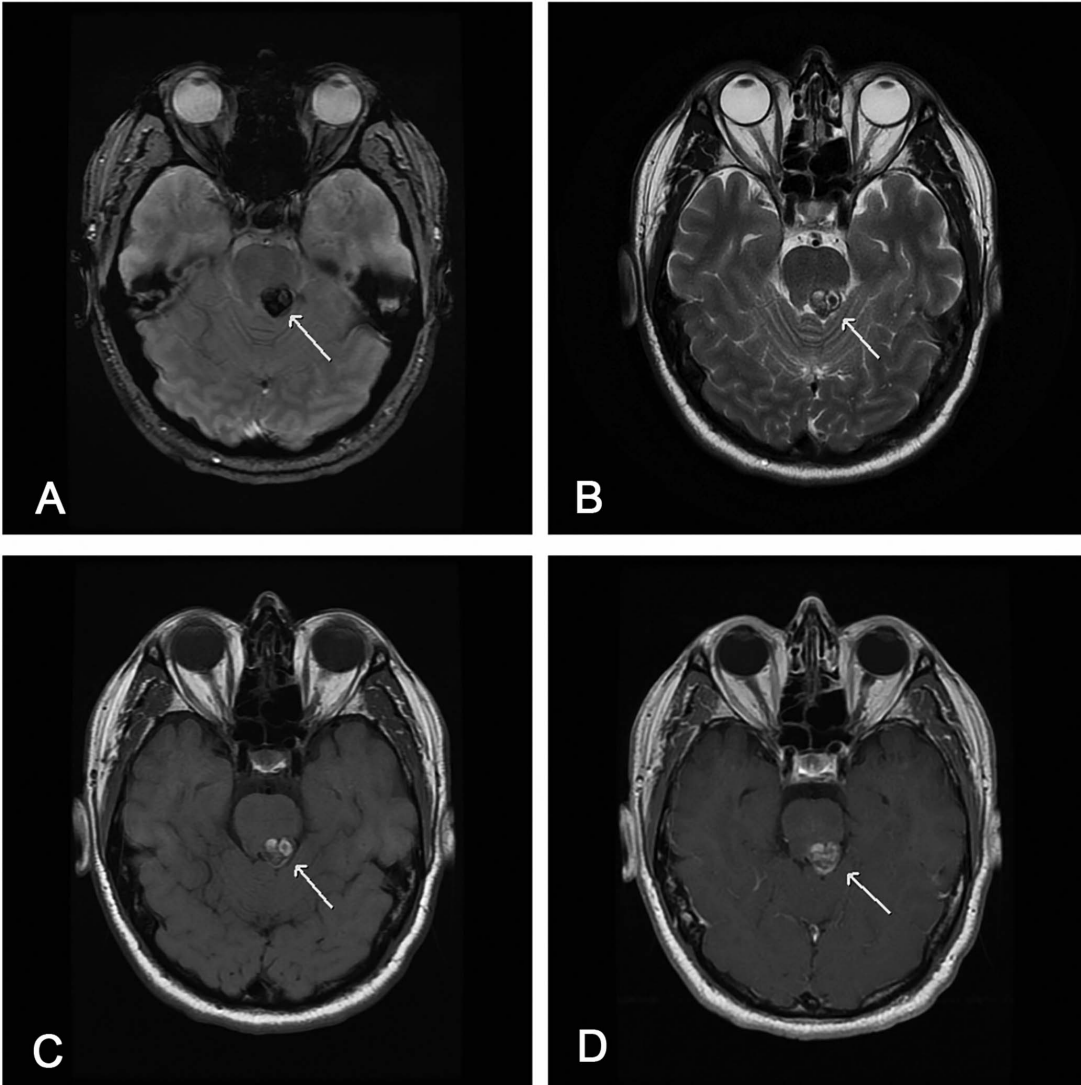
Dorsal midbrain cavernous malformation demonstrating classic popcorn appearance. Axial T2 gradient echo image (A) shows prominent susceptibility artifact indicating blood products (arrow). Axial T2-weighted image (B) depicts heterogeneous signal centrally with hypointense hemosiderin rim (arrow). No surrounding edema. T1-weighted images before (C) and after (D) intravenous contrast administration show minimal postcontrast enhancement of the lesion (arrows).
